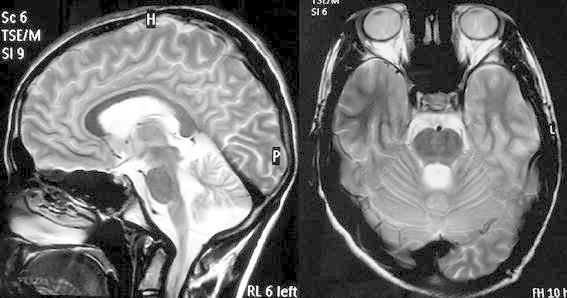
Magnetic resonance imaging (MRI) of brain showing cerebellar atrophy.
